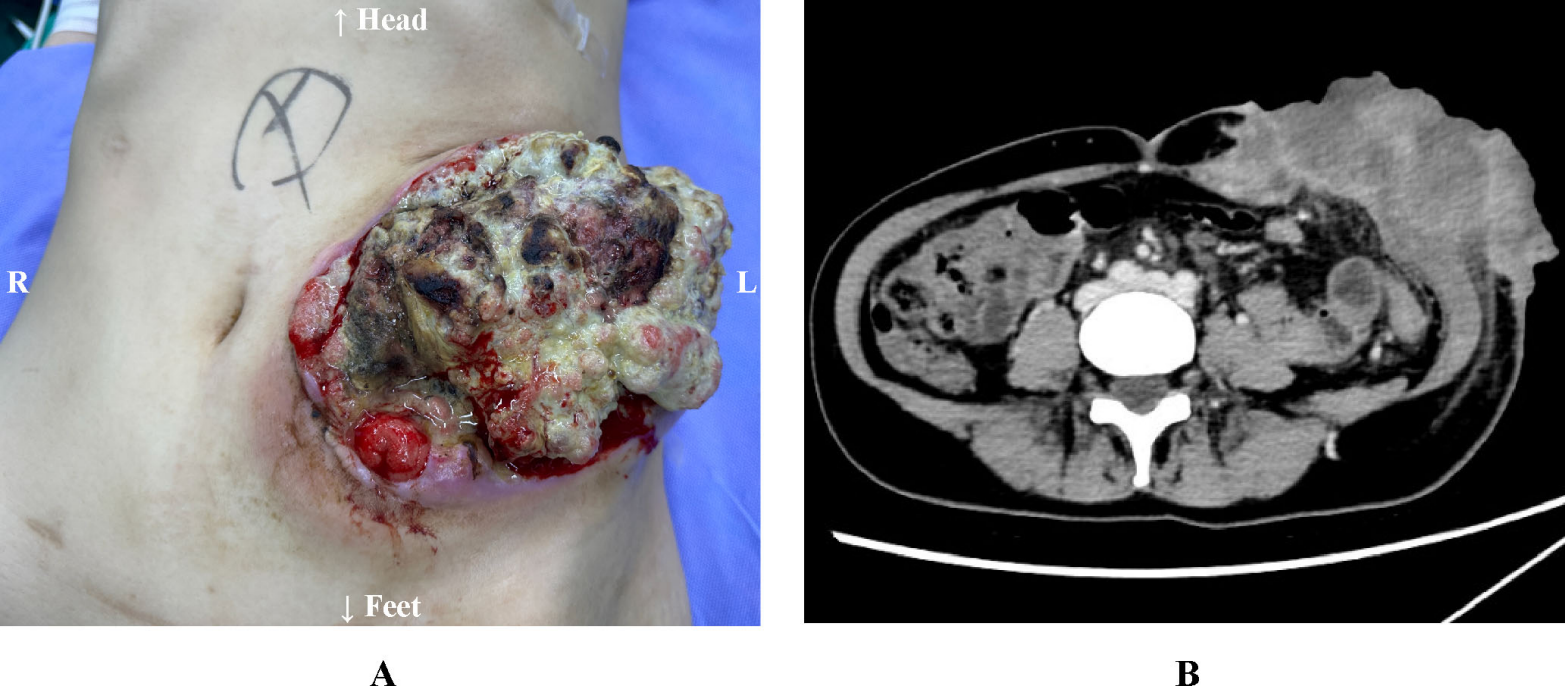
(A) The large tumor (approximately 10×10 cm) is associated with necrosis and hemorrhage. (B) Abdominal CT Scan findings (Preoperative, February 2025).
